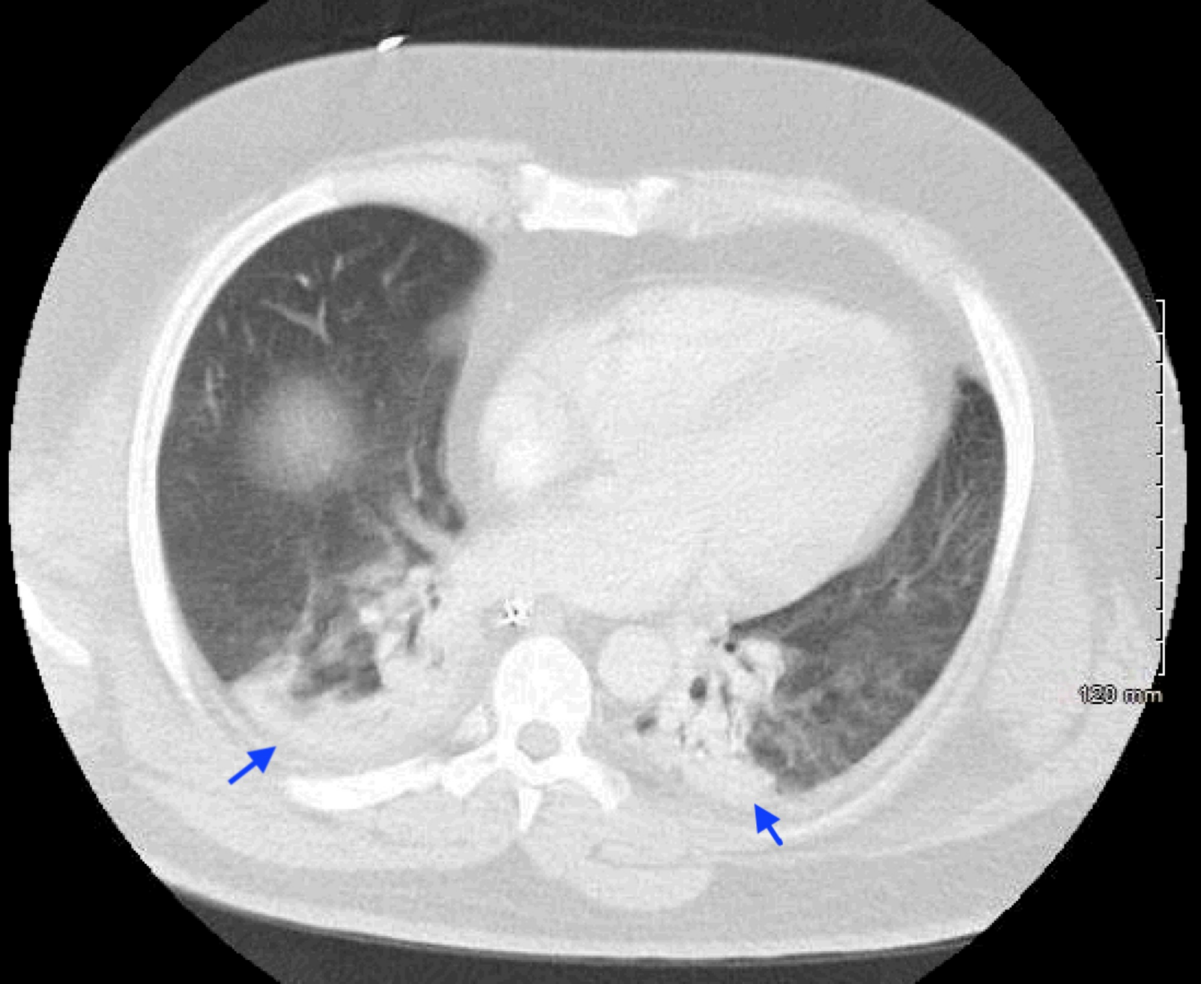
CT chest with bilateral lower lung consolidations
